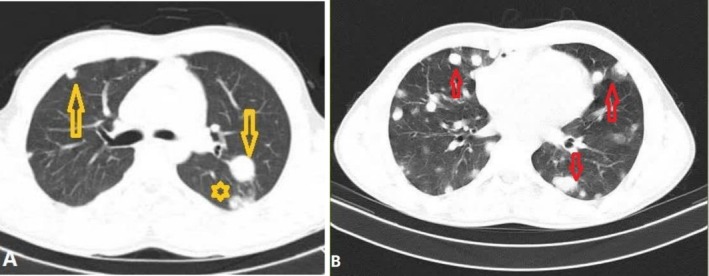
Preoperative contrast‐enhanced chest CT in the lung window demonstrates bilateral solid nodular lesions (yellow arrows) accompanied with surrounding ground‐glass opacity (asterisk) (A). A post‐treatment contrast‐enhanced chest CT obtained 10 months after the initiation of chemotherapy shows multiple pulmonary metastases (red arrows) (B).
